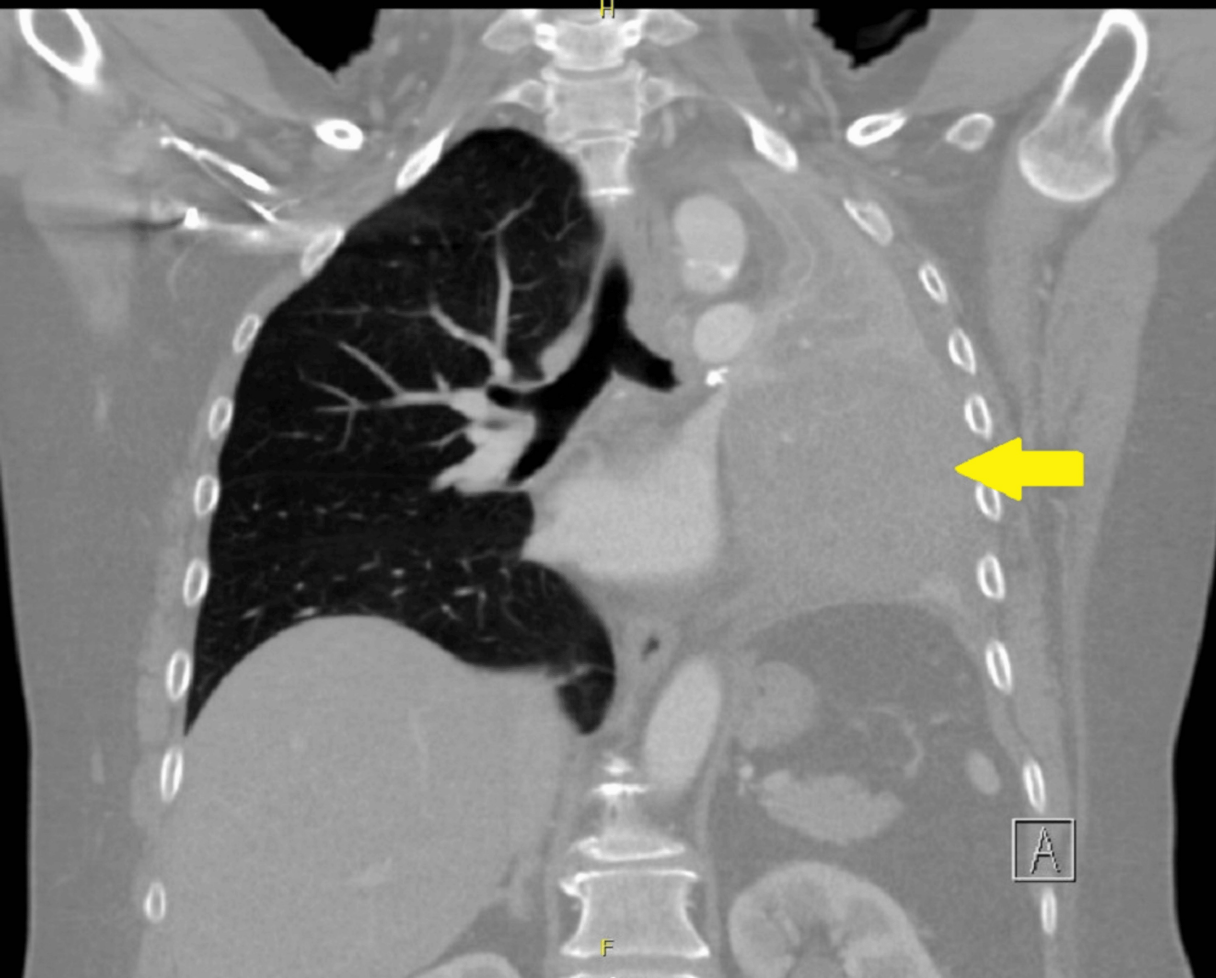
CT of the chest with heterogeneous mass-like consolidation in the left upper lobe (yellow arrow) and total occlusion of the left upper and lower lobe bronchi.
